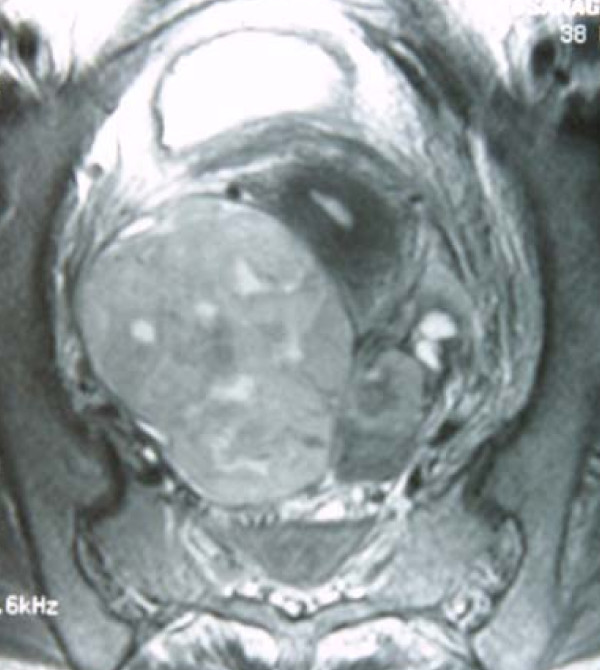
Magnetic resonance image of the right ovarian tumor on week 27 post-transplantation.
